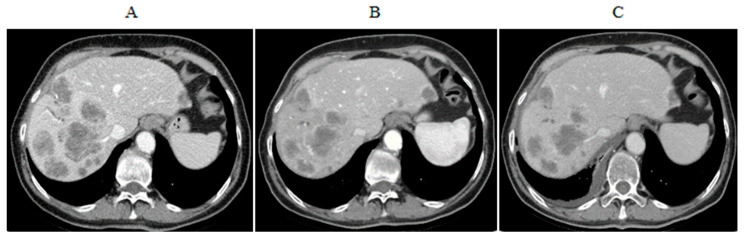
CT scans of the European Institute of Oncology (EIO) responding patient with liver metastasis from iCCA included in the phase II trial. She received two cycles of CS-PHP and achieved a partial response with reduction in the number and dimension of the lesions. Pictures show (A) pretreatment, (B) after first PHP and (C) after second PHP.
